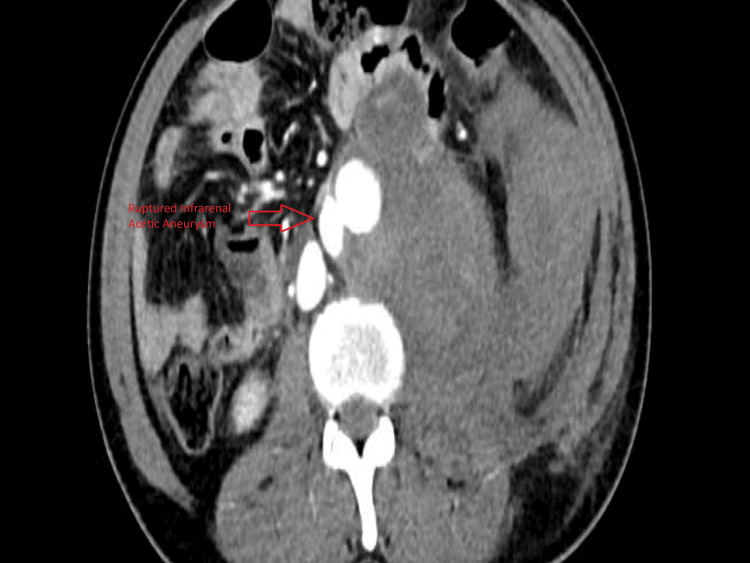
CT scan showing contrast extravasation in infrarenal abdominal aorta
